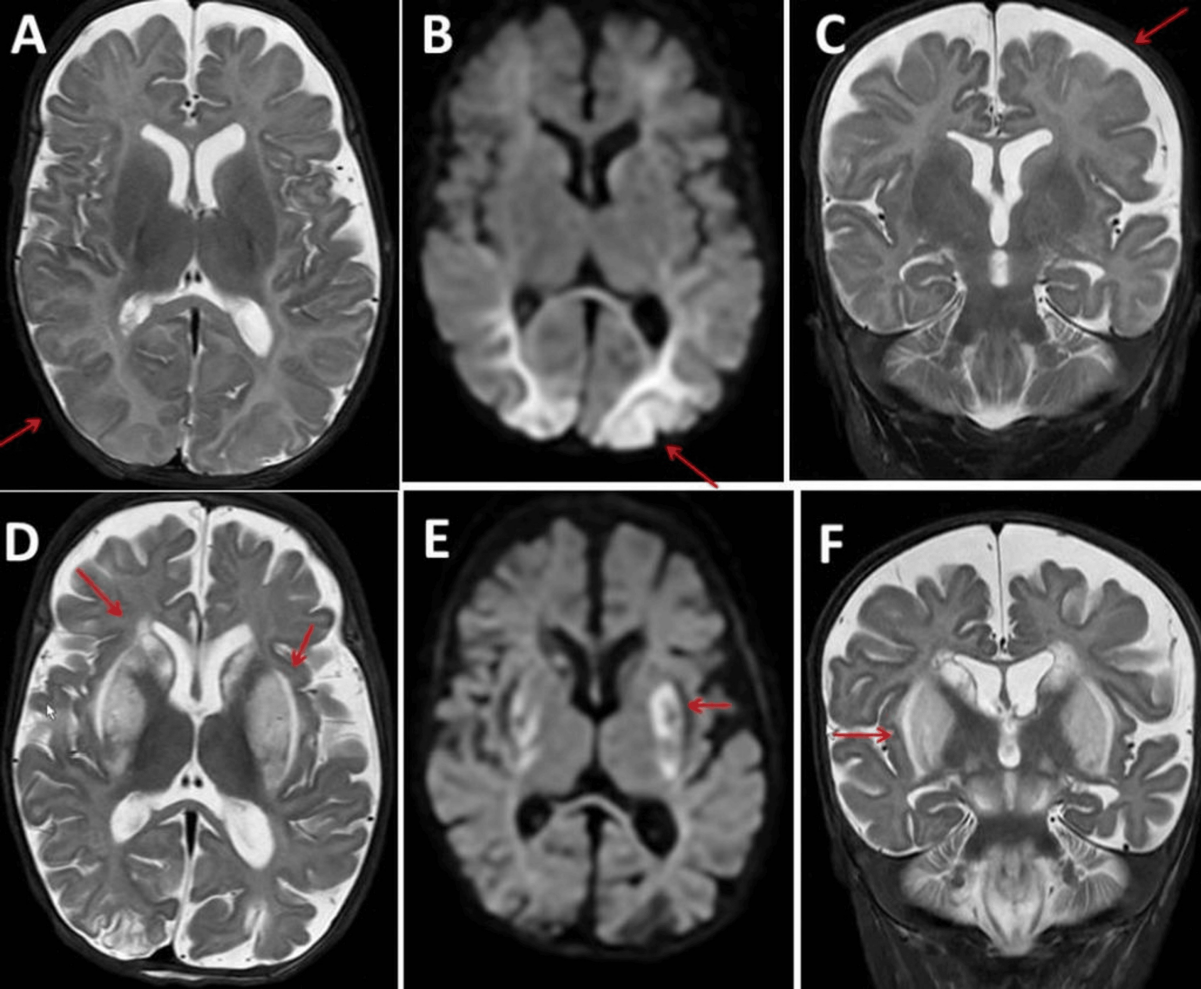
Cerebral magnetic resonance imaging shortly after admission (panels A to C) compared with images from four weeks later (panels D to F) showing progression of axial and coronary T2 hyperintensities in the basal ganglia (panels A and D and panels C and F) and new diffusion-weighted imaging (DWI) hyperintensities in the basal ganglia (panel E) but regression of DWI hyperintensities in the occipital lobes (panel B)
